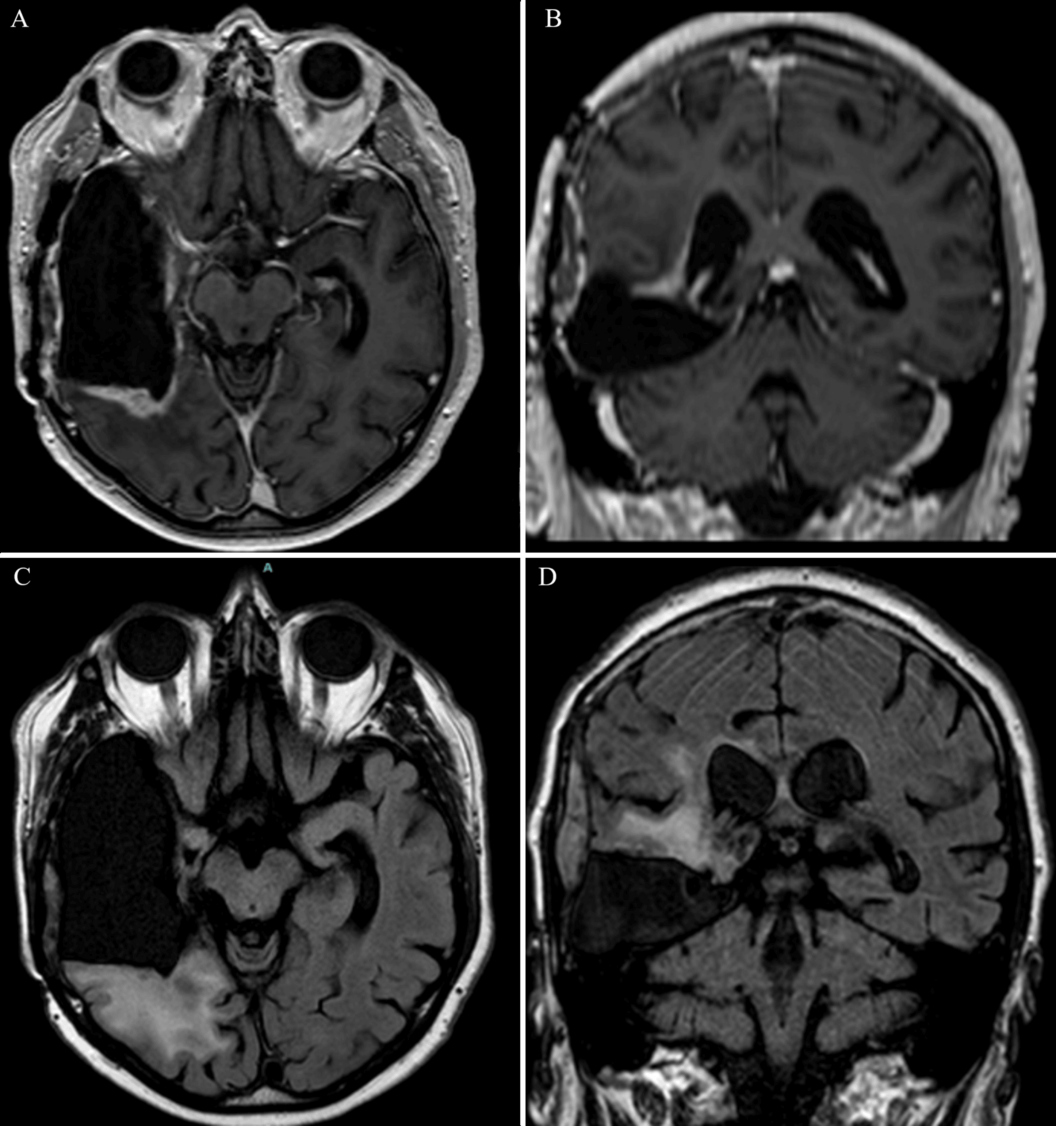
Axial (A) and coronal (B) T1-weighted MRI with contrast, along with axial (C) and coronal (D) FLAIR sequences at the time of second recurrence, showing increased enhancement along the posterior aspect of the patient’s resection cavity
